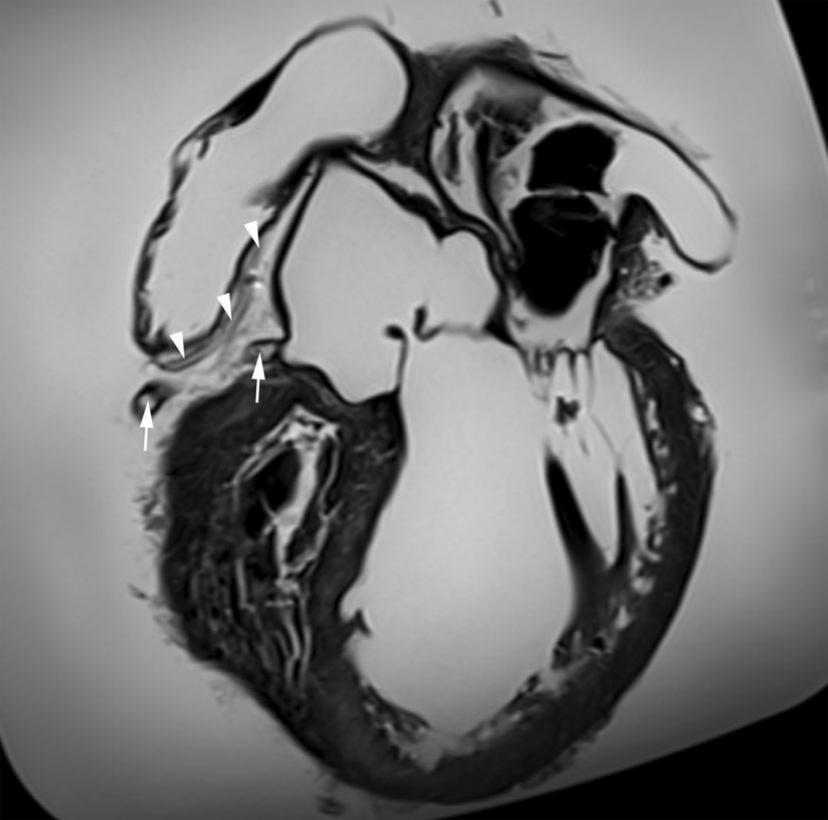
Magnetic resonance imaging (from T2-weighted sequences) showing part of the course of the RCA and its atrial branch. The white arrows indicate the RCA and the white arrowheads the atrial branch of the RCA
